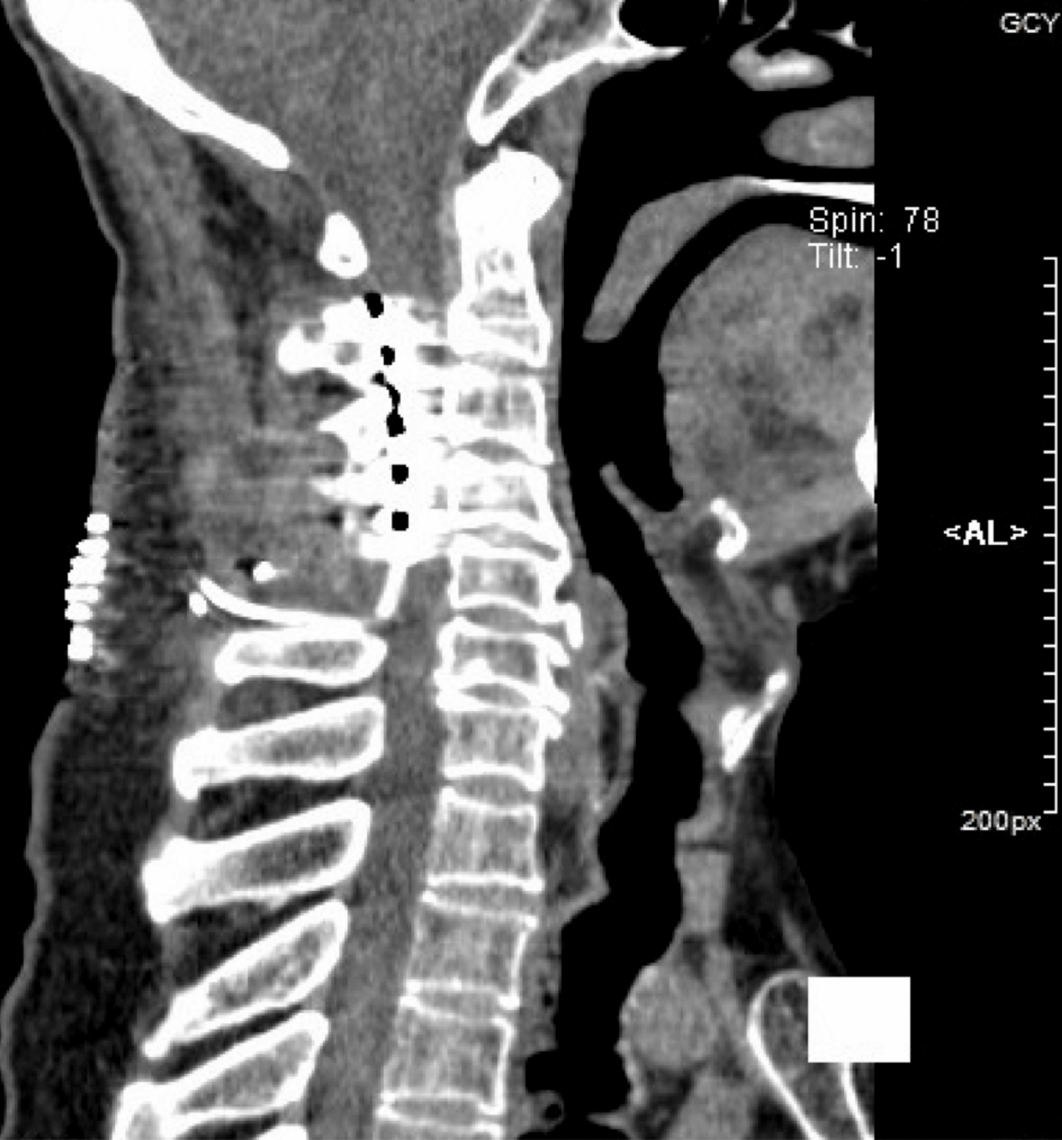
A postoperative review of the neck CT scan in the sagittal section reveals that a spinal cord stimulator was implanted at the C2 to C4 levels
